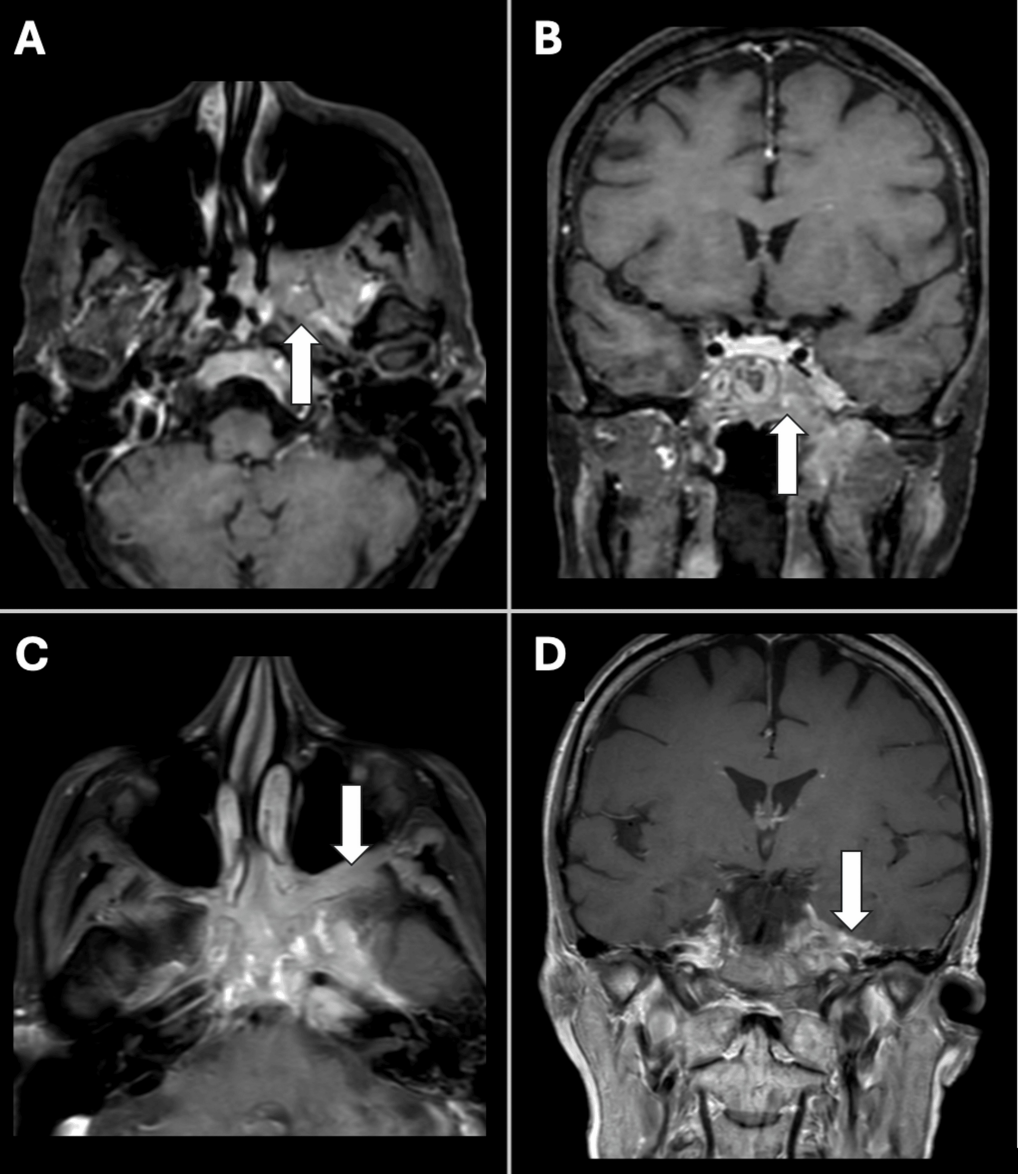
Initial contrast-enhanced MRI of the head T1-W images demonstrating an enhancing mass centered on the body of the left sphenoid bone (A, B). Extensive soft tissue perineural extension is observed, obliterating the left pterygopalatine fossa and extending anterolaterally along the posterior wall of the maxillary antrum (C). Additionally, there is direct invasion through the skull base with dural infiltration along the medial floor of the middle cranial fossa (D).
